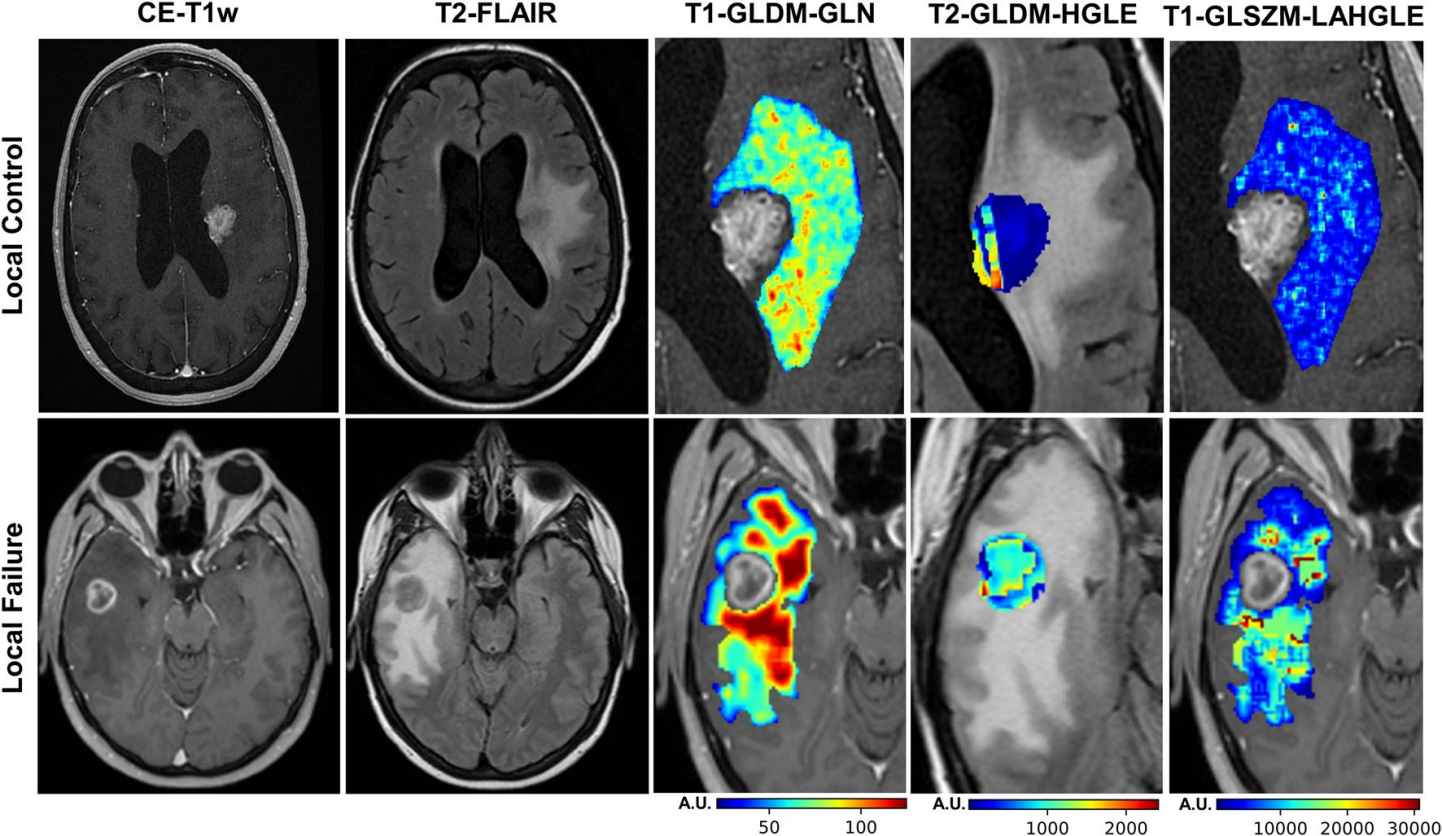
CE-T1w and T2-FLAIR images and parametric maps of the texture features in the optimal quantitative MRI biomarker for two representative lesions with LC and LF outcomes after SRT.
